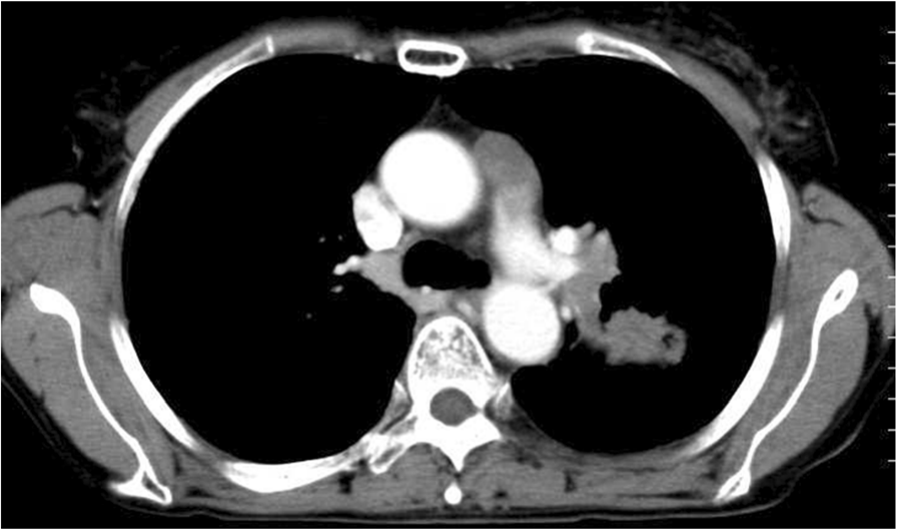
Preoperative computed tomography. Hilar lymph nodes infiltrating the pulmonary artery, and mediastinal lymph node (#6) and main tumor of S1 + 2 segments
